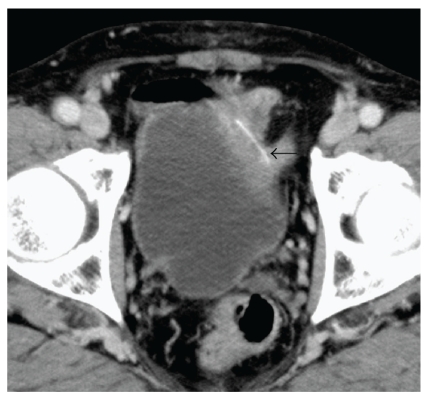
CT scan showed a linear radiopaque structure (*arrow*) traversing the thickened anterolateral wall of the bladder.
